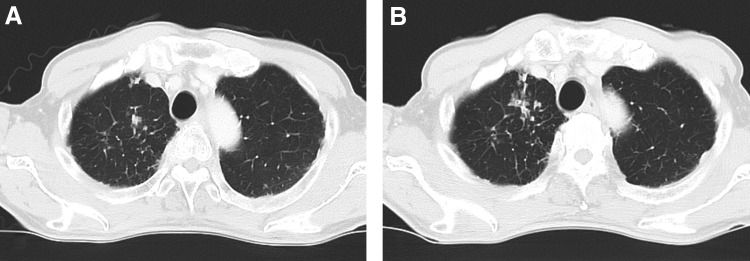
CT of the left upper lung: (A) preoperative and (B) postoperative. The granular shadows and a mucous plug indicate an old TB infection. TB, tuberculosis.
